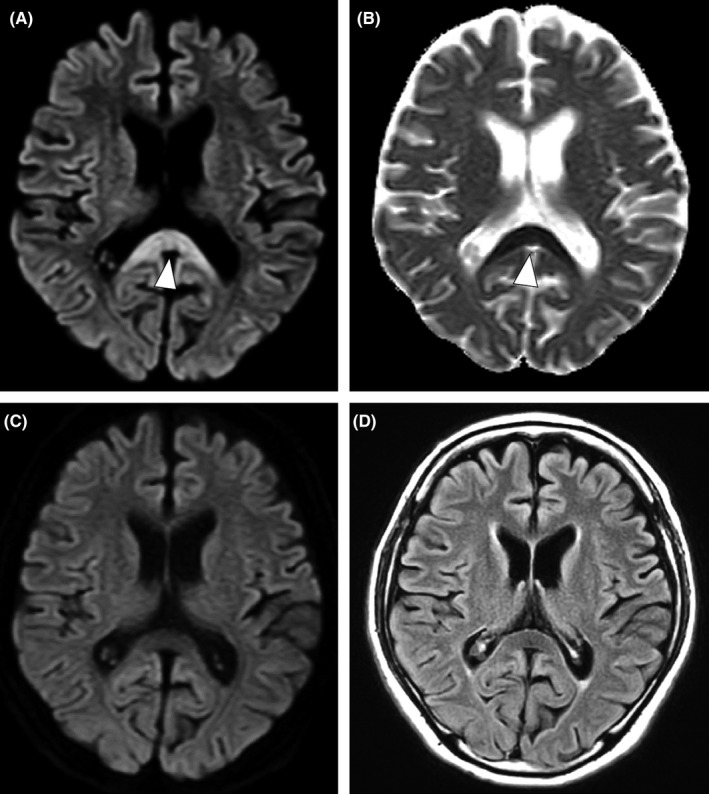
(A and B); MRI studies at onset. Diffusion‐weighted images (DWI) show hyperintensity involving the splenium of the corpus callosum (arrowhead) (A) with corresponding hypointensity on apparent diffusion coefficient (ADC) images (B). (C and D); MRI studies at two‐week follow‐up. DWI shows normal signal in the splenium of the corpus callosum (C). There is no abnormal lesion on fluid‐attenuated inversion recovery (FLAIR) (D)
